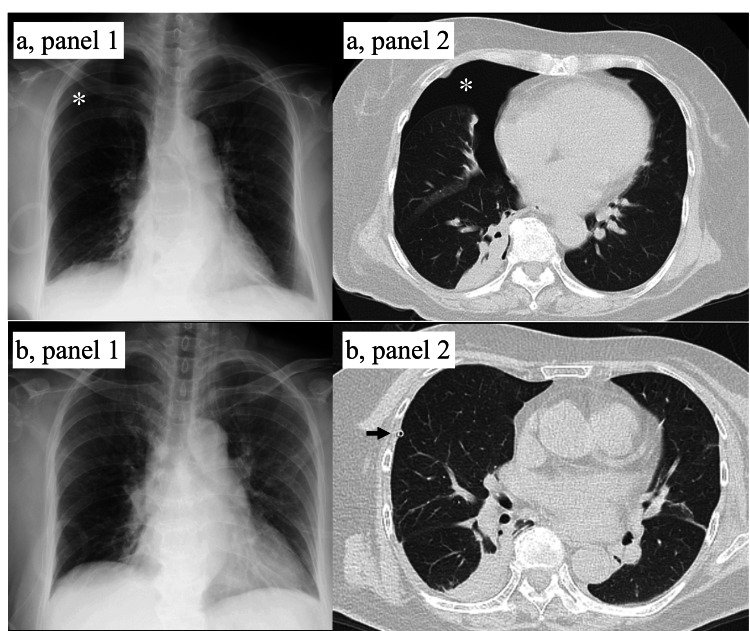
Radiological findings of pneumothorax (a) Before drainage. (a, panel 1) Chest radiograph on POD2 showing right pneumothorax (asterisk). (a, panel 2) Chest CT scan on POD2 confirming right pneumothorax (asterisk) without subcutaneous emphysema. (b) After chest tube drainage. (b, panel 1) Chest radiograph showing full lung expansion. (b, panel 2) Chest CT scan demonstrating re-expansion of the right lung (arrow: chest tube).
